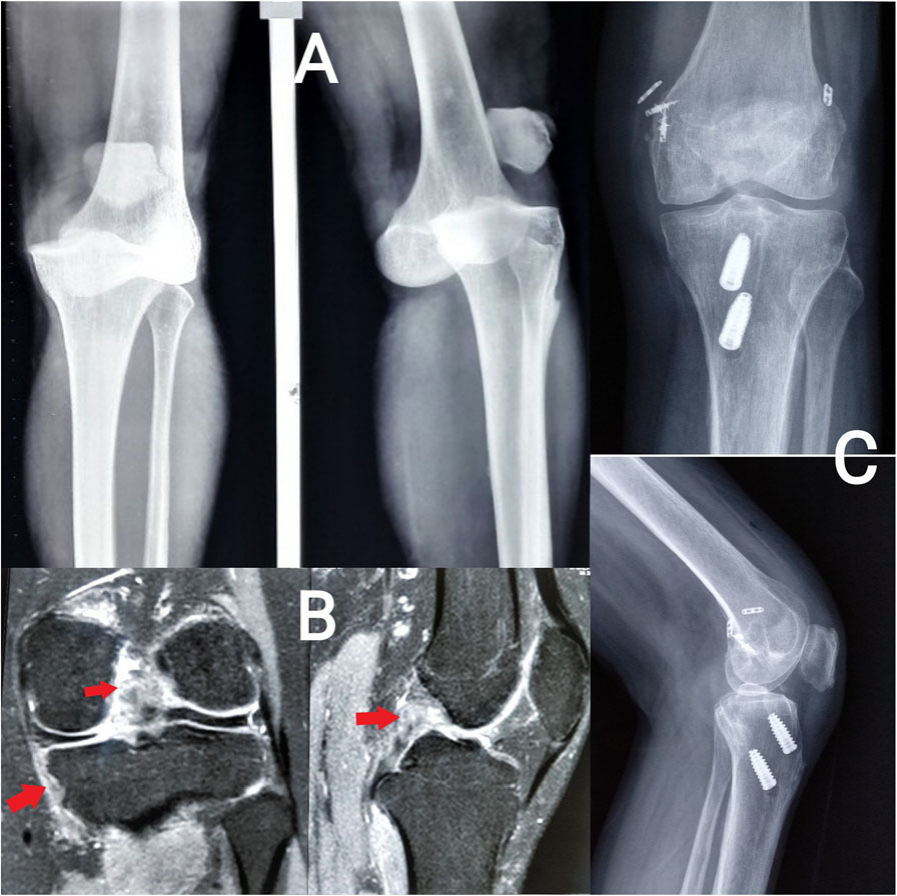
a Radiographs before reduction of the knee joint. b Fat-suppressed coronal and sagittal MRI images of a patient with ACL, PCL, and MCL tears. c For patient with a KD type III M injury, postoperative radiograph after ACL, PCL reconstruction and MCL repair
